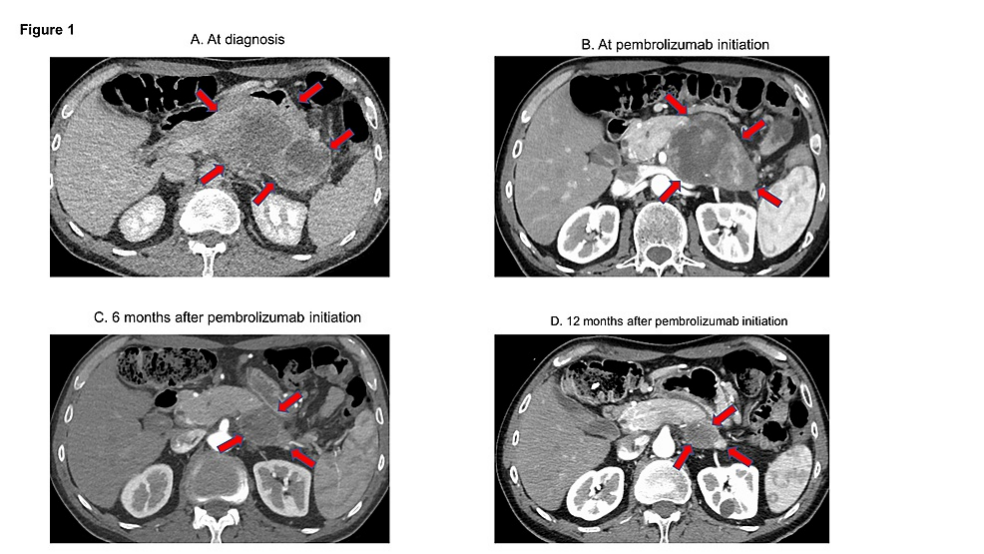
Representative CT images of pancreatic mass at diagnosis and after treatment (A) At diagnosis, the pancreatic body and tail were occupied by a large heterogeneous solid and cystic mass measuring 11.6 x 6.4 cm. Invasion into the surrounding vasculature was noted. (B) About three months into the initial treatment (hyperthermia with low-dose chemotherapy), the pancreatic mass size decreased, measuring 7.9 x 5.9 cm. Pembrolizumab was started at this time. (C) The pancreatic mass demonstrated further shrinkage measuring 5.3 x 3.7 cm, about six months after the first dose of pembrolizumab.  (D) Approximately 12 months after the first dose of pembrolizumab, the pancreatic tumor showed markedly decreased size, measuring 3.1 x 3.0 cm.
